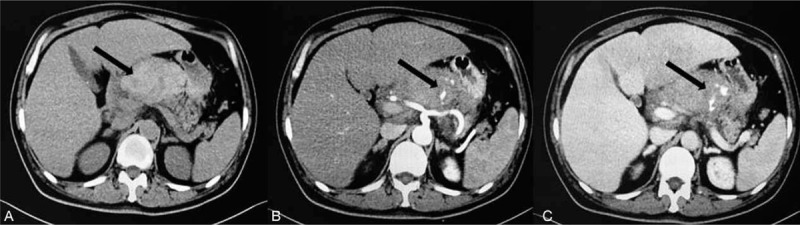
Enhanced CT scans of the patient before intervention. (A) Black arrow shows a 12 cm hematoma near the head of pancreas and duodenum. (B) Artery phase, black arrow shows extravasation of the contrast medium. (C) Vein phase, black arrow shows the enlargement of extravasation of the contrast medium. CT = computed tomography.
